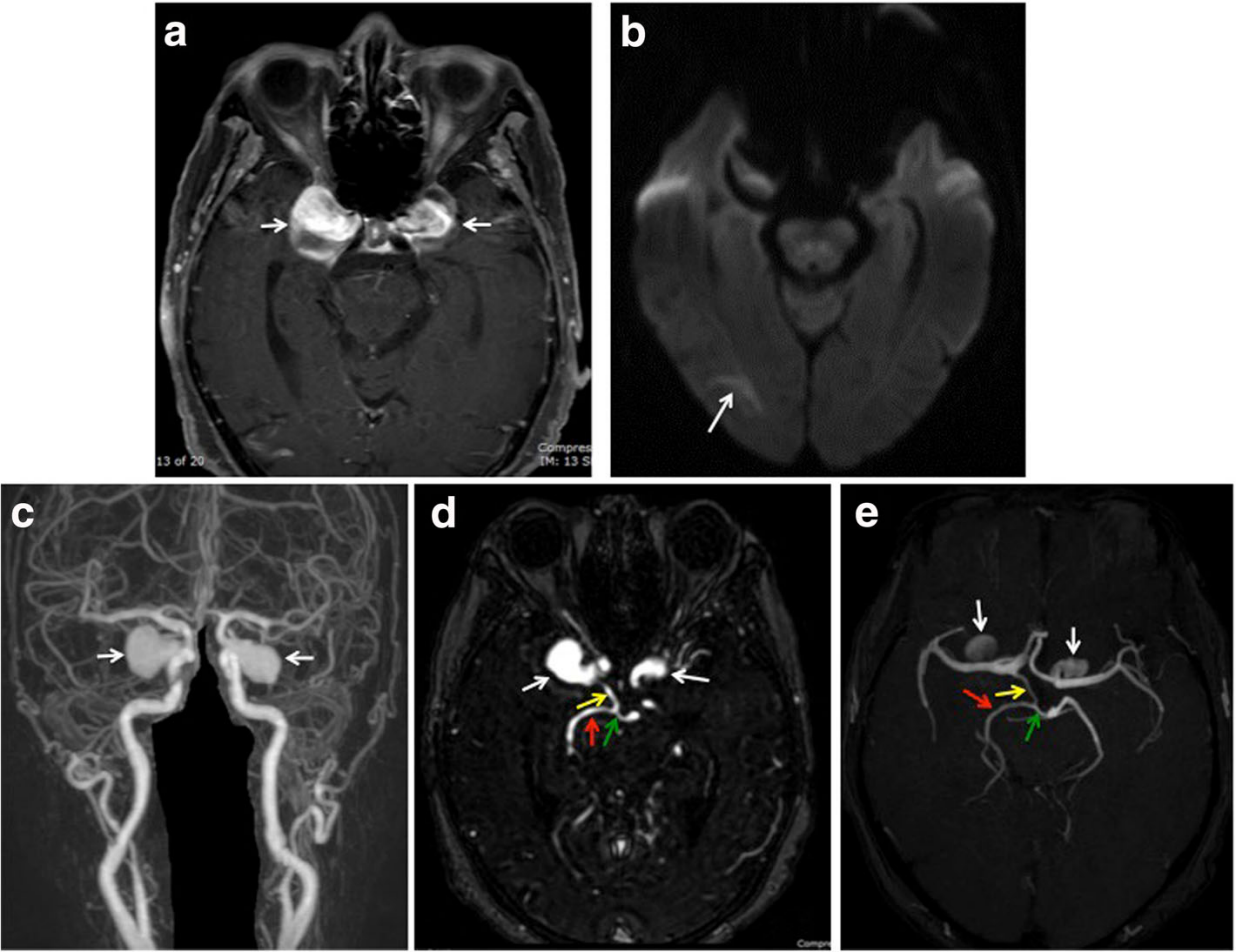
Magnetic resonance imaging (MRI) and magnetic resonance angiography (MRA) of the brain. a Contrasted MRI: partially thrombosed bilateral giant cavernous carotid aneurysms (CCAs, arrows). b Diffusion-weighted MRI: restricted diffusion of the right occipital lobe (arrow). c–e MRA: bilateral CCAs (white arrows) and dual vascular supply of right posterior cerebral artery (PCA, red arrows) from prominent posterior communicating artery (PCOM, yellow arrows) and P1 (green arrows). Left PCOM is absent
